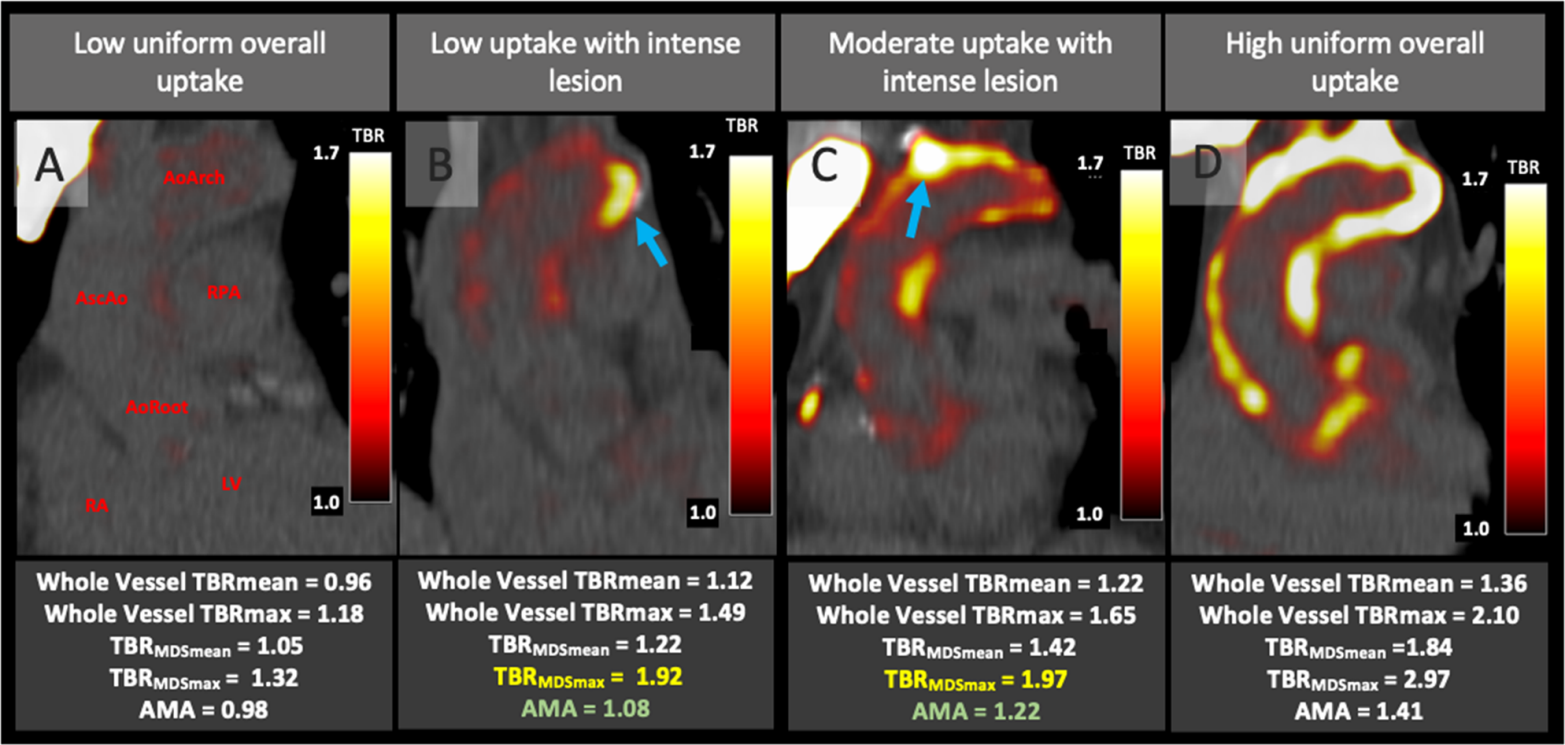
Hybrid ^18^F-sodium fluoride positron emission tomography and computed tomography coronal images of the ascending aorta and arch in four patients with varying patterns and intensity of aortic wall ^18^F-sodium fluoride activity: (A) Homogenously low activity across the ascending aorta and arch; (B) Generally low activity with a single high intensity lesion (blue arrow); (C) Moderate activity with a high intensity lesion (blue arrow); (D) High and intense activity throughout ascending aorta and arch. Note that (B) and (C) have similar values for most diseased segment maximum tissue to background ratio (highlighted in yellow) despite substantially different overall activity (aortic microcalcification activity values highlighted in green). *AscAo*, ascending aorta; *AMA*, aortic microcalcification activity; *AoArch*, aortic arch; *AoRoot*, aortic root; *MDS*, most diseased segment; *LV*, left ventricle; *RA*, right atrium; *RPA*, pulmonary artery; *SUV*, standardised uptake measurement; *TBR*, tissue to background ratio
